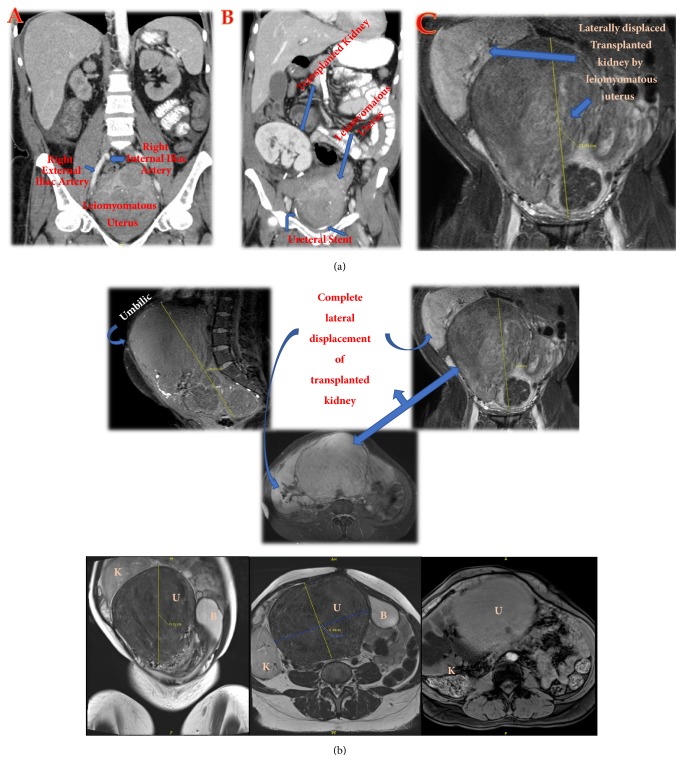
(a) Abdomen/pelvis CT scan and MRI showing progressive enlargement of the leiomyomatous uterus with time: pretransplant (A-12.2cms), immediately posttransplant (B-12.2 cms), and 4 years after transplant (C– 21.6 cms). Note: A=8/11/2012, B=12/12/2012, and C=11/01/2017. (b) Abdomen/pelvis MRI depiction of massively enlarged leiomyomatous uterus (U) completely replacing the urinary bladder (B) and transplanted kidney (K), causing severe hydronephrosis. Note that the uterus fundus is extending 5 cms above the umbilicus.
